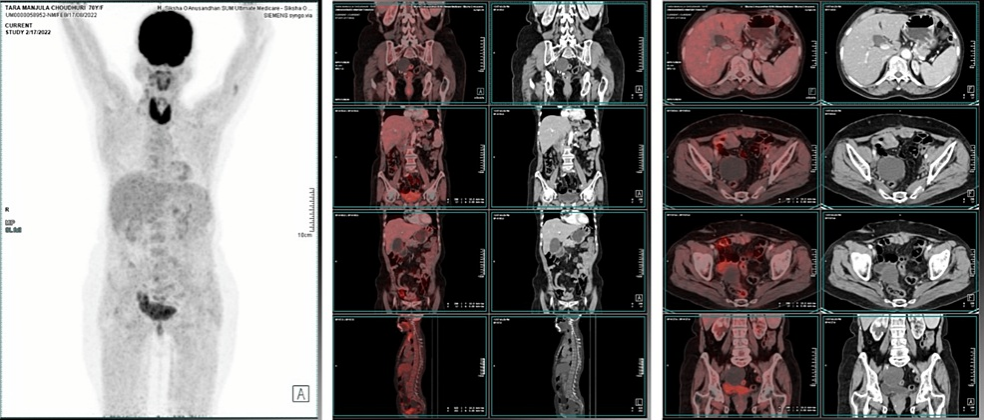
The PET/CT shows a complete response to rucaparib at the sixth-month follow-up PET/CT: Positron emission tomography-computed tomography
